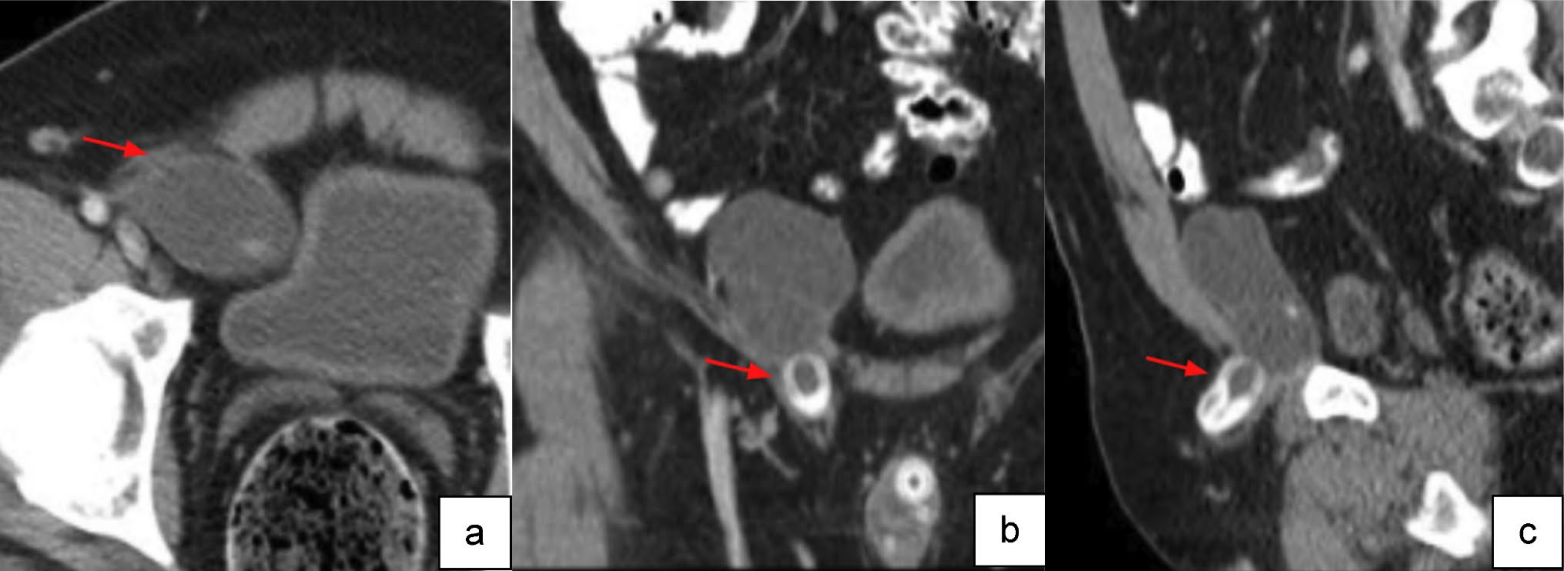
Axial (a), coronal (b) and sagittal (c) CT images with IV contrast show IPP reservoir partially extending into the inguinal canal (red arrows)
